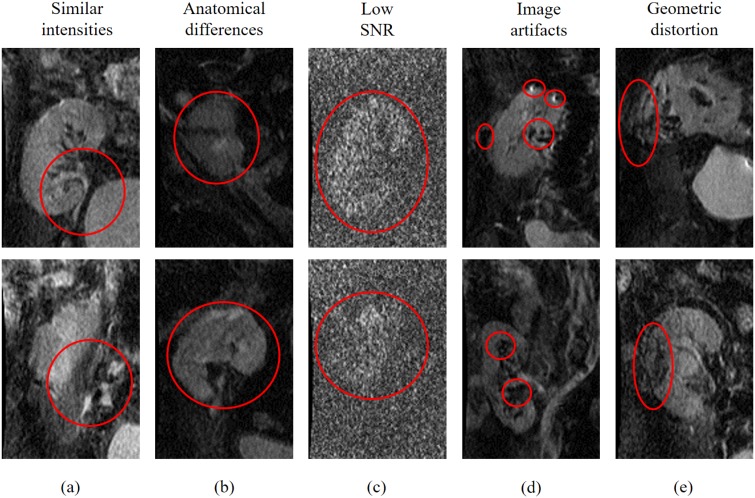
Typical coronal cross-section DW-MRI samples showing (a) low contrast between the kidney and surrounding abdominal tissues at *b*_0_; (b) inter-patient anatomical differences at *b*_0_, (c) low signal-to-noise ratio (SNR), especially, at higher *b*-values (e.g., *b*_1000_); (d) image artifacts; and (e) geometric distortion/diffused boundaries.
